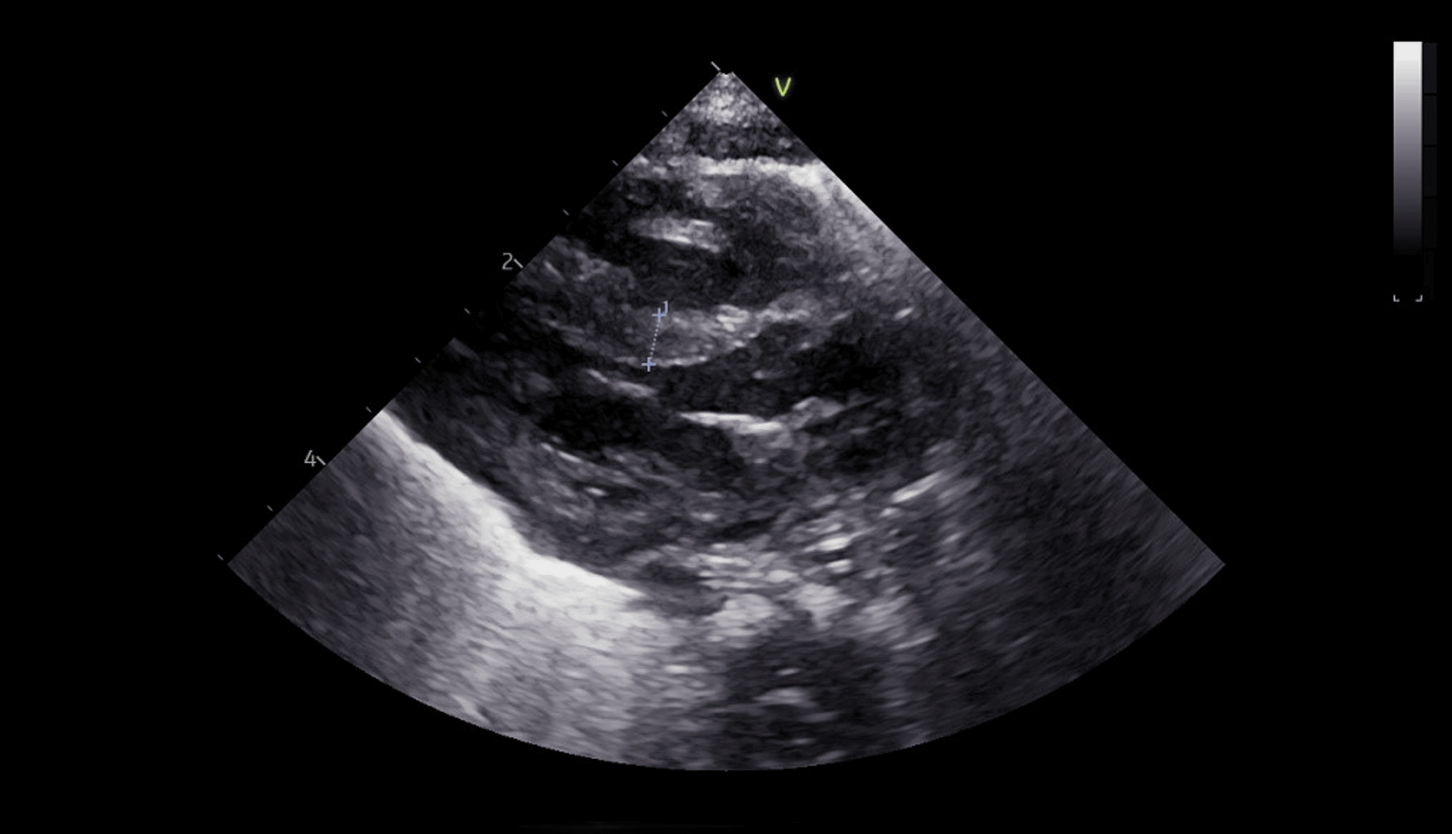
Echocardiography: parasternal long-axis view showing left ventricle hypertrophy
